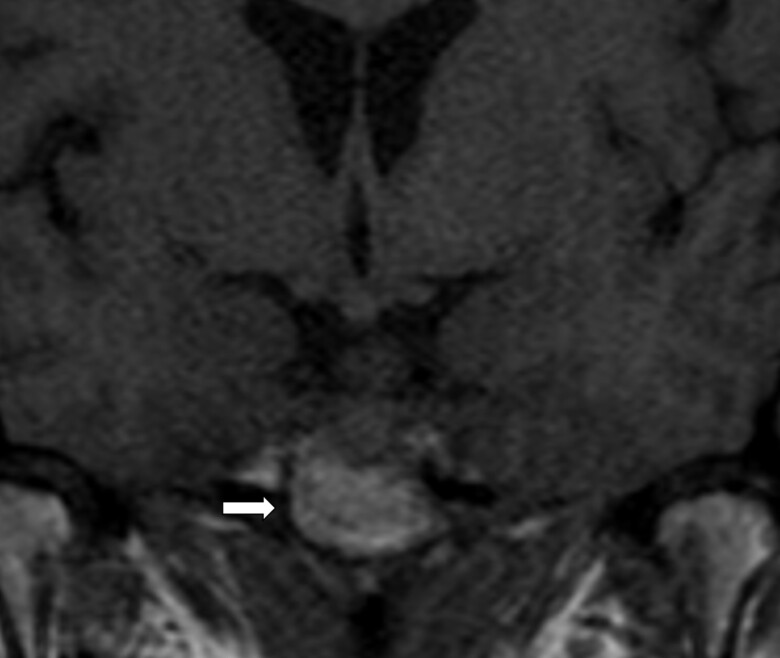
Magnetic resonance imaging of the brain: 1.8 cm sellar mass (arrow) causing deviation of the optic chiasm.
